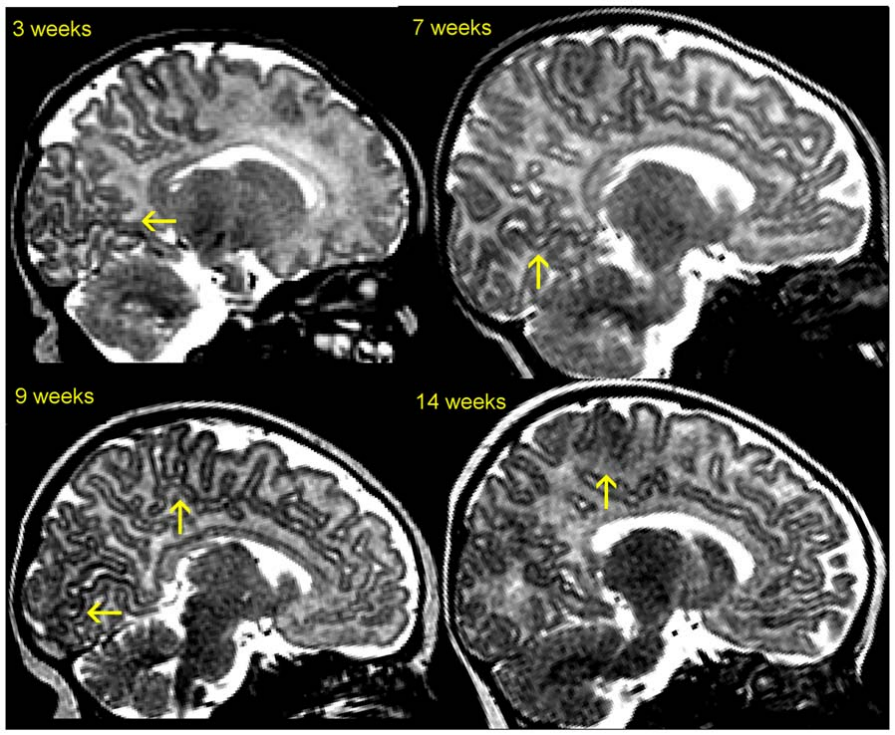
Infant brain maturation across age in T2w MRI. These sagittal slices show temporo-spatial variations of the on-going maturation processes near the inter-hemispheric plane. Areas of advanced maturation can be seen in primary cortices, such as along the calcarine sulcus and the central sulcus (*yellow arrows*).
